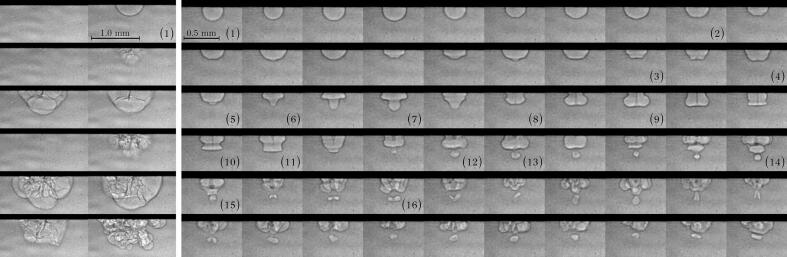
X-ray imaging of the inception and dynamics of single cavitation bubbles at $P_{h}$ = 30 %. The dark region at the top is the tip of the horn, so that acoustic radiation is from top to bottom. The time of frame (1) on the left and right panel is 51.6 ms and 35.5 ms, respectively. The interframe time is 12.5 μs. Note that the transient regime of the ultrasonic horn lasts ≈ 16 ms, after which the full field is established. The Feret diameter of the bubble on frame (1) of left and right panels is 560 μm and 300 μm, respectively. Left and right panels are images from two different experiments.
